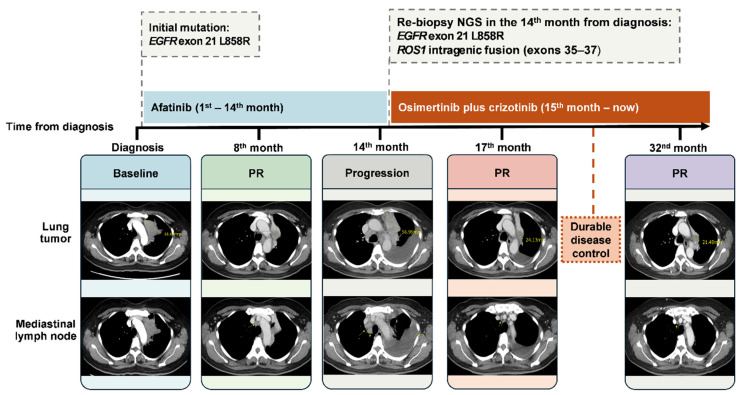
Timeline of Case 1 with durable response to dual *EGFR*-*ROS1* inhibition in *EGFR*-mutant NSCLC with acquired *ROS1* rearrangement. Serial chest CT images show changes in the target lung lesion and mediastinal lymph nodes. The lung tumor measured 62 mm at diagnosis, decreased to 29 mm at month 8, increased to 57 mm at progression at month 14, and decreased to 24 mm and 21 mm after osimertinib plus crizotinib at months 17 and 32, respectively. Target lesion measurements and response assessments are shown according to RECIST v1.1 where evaluable. PR: partial response; NGS: next-generation sequencing.
